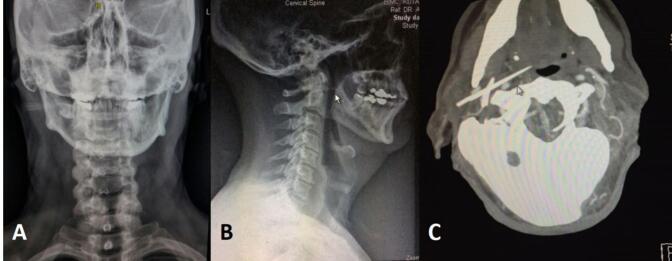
A. Skull AP view; B. Skull lateral view; C. Head CT scan.
